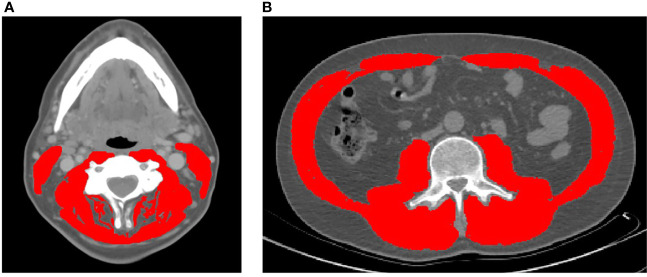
Representative axial CT images of the third cervical (A) and third lumbar (B) vertebral levels used to quantify skeletal muscle index (highlighted in red).
